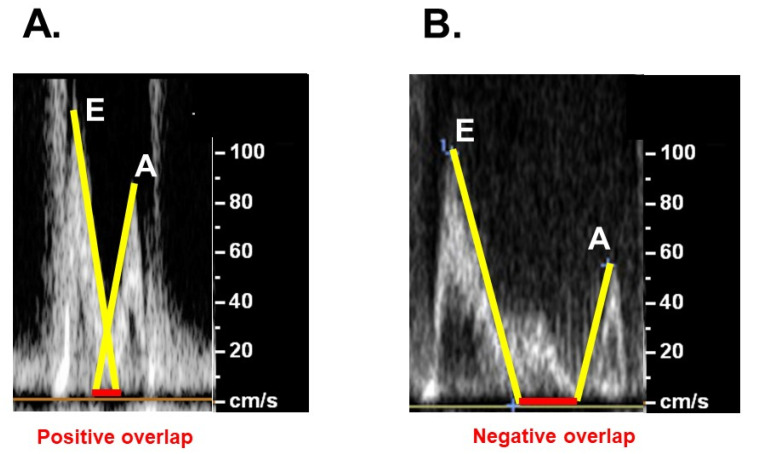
Trans-mitral inflow velocity focusing on the overlap between E-wave and A-wave using Doppler echocardiography on admission (A) and following heart rate modulation therapy using ivabradine (B). The two waves were overlapped in (A) and completely apart in (B).
